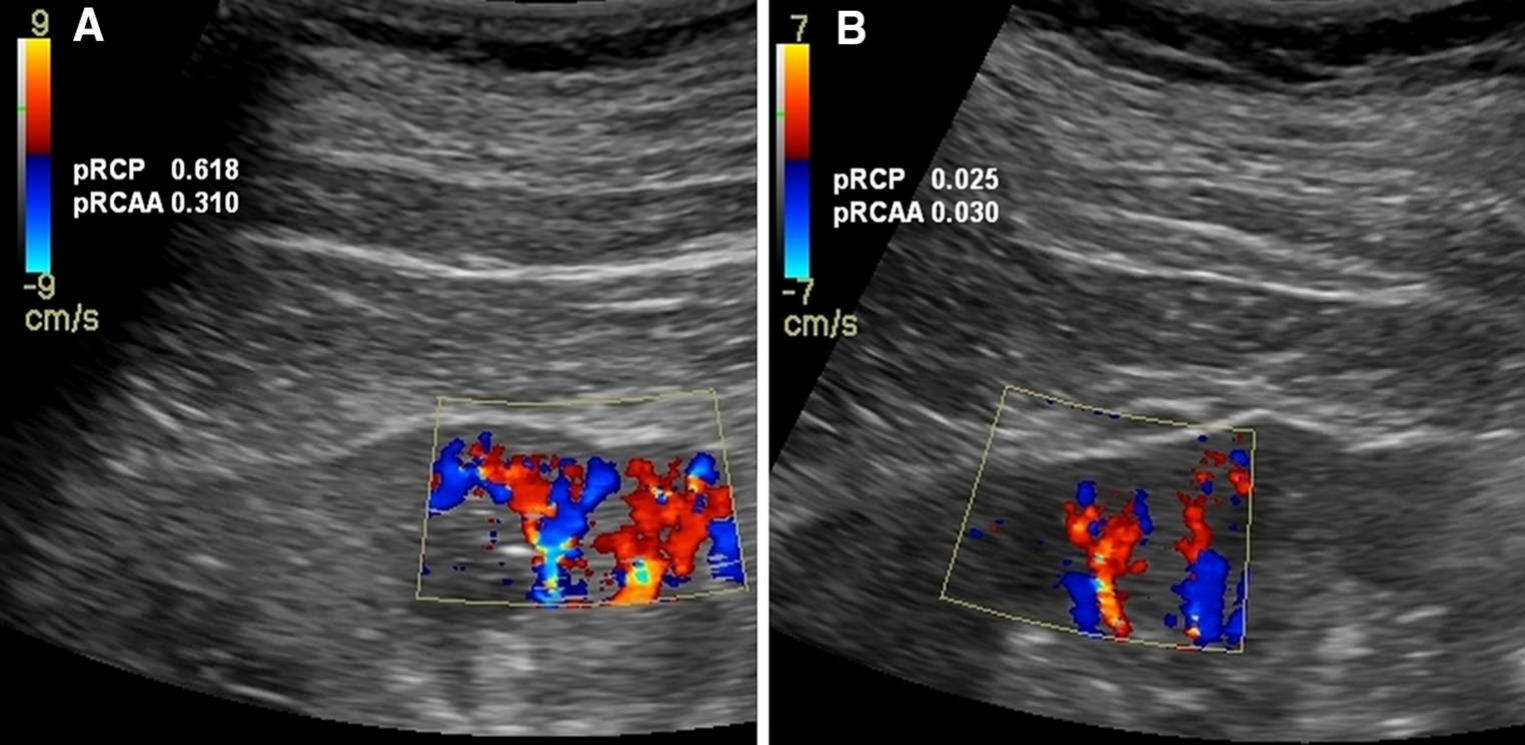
Color Doppler imaging of renal cortical perfusion. Right kidney cortical perfusion imaging: a 61-year-old man with GN-CKD; b 60-year-old man with HT-CKD
